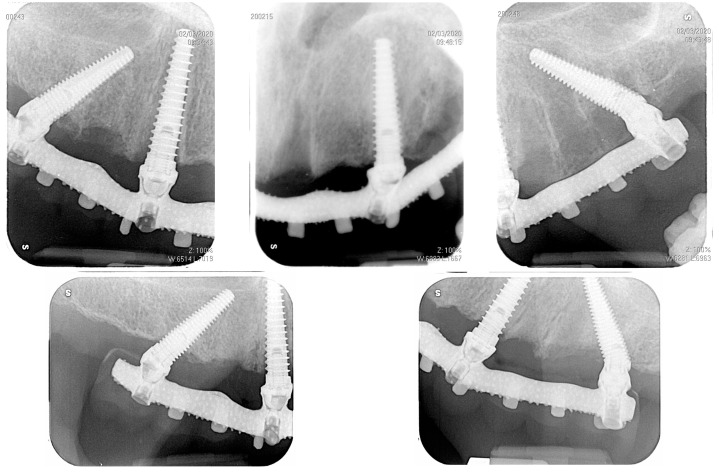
Intraoral radiographs taken at the delivery of the immediate loading prosthesis (T0) and after 24 months in the same patient of Figure 1.
